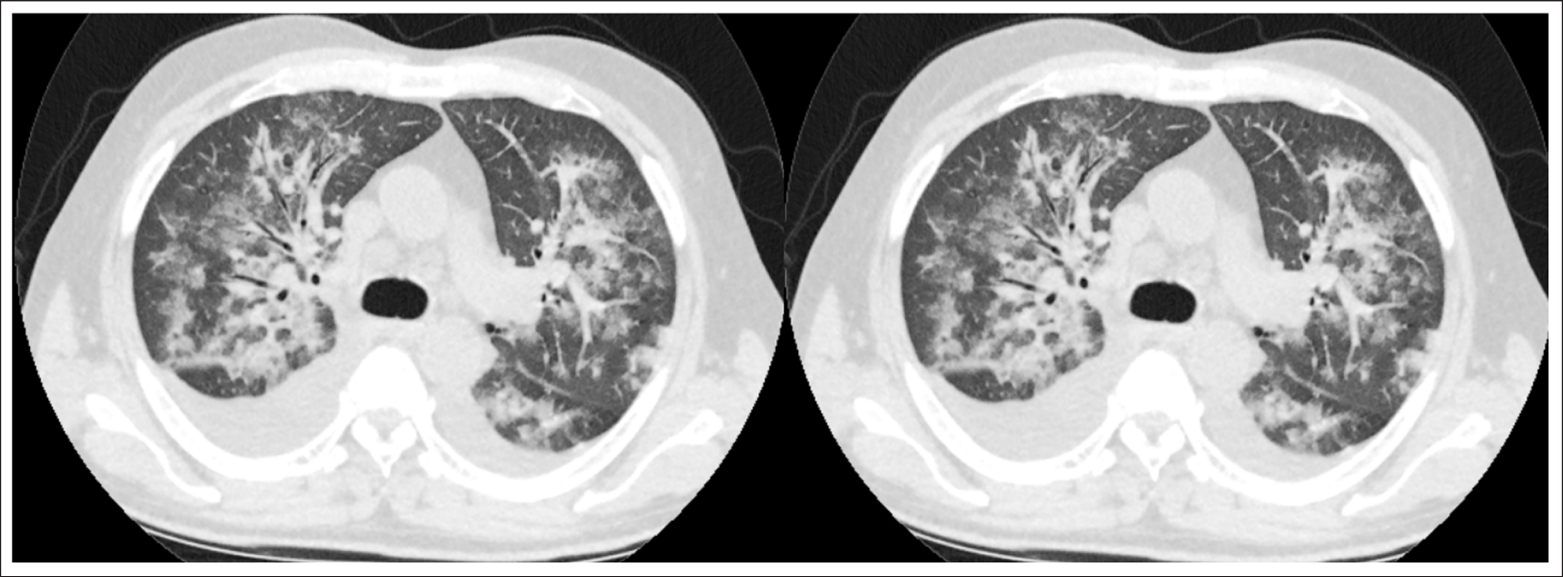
RT-PCR –, CO-RADS 5: This 56-year-old male patient had no comorbidity other than coronary artery disease. He presented to the emergency department with a fever of 38.4 degrees. He had no respiratory symptoms. There were no findings compatible with COVID-19 in laboratory parameters. RT-PCR test was negative, multifocal areas of ground glass and consolidation with bilateral pleural effusion were seen on chest CT taken in his emergency admission, and he was diagnosed with COVID-19 and hospitalized. It was evaluated as CO-RADS 5. PCR tests taken on the 1st, 5th, and 11th days of his hospitalization are also negative. The need for intensive care developed on the 11th day of his hospitalization, and he died on the 17th day.
